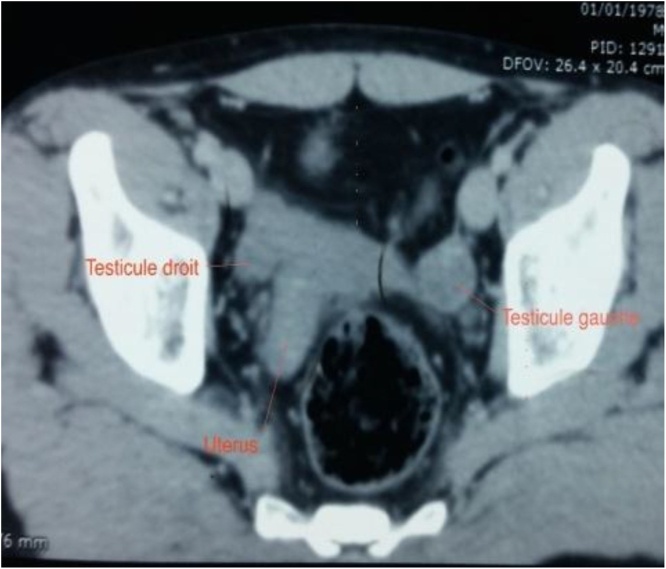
Pelvic CT showing uterus and two intra-abdominal testicles.
